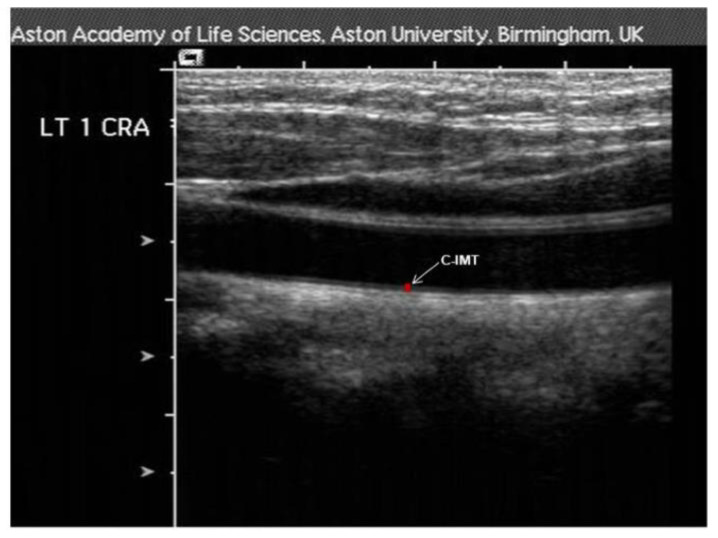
Ultrasound image of carotid intima-media measurement site. C-IMT: carotid intima-media thickness.
